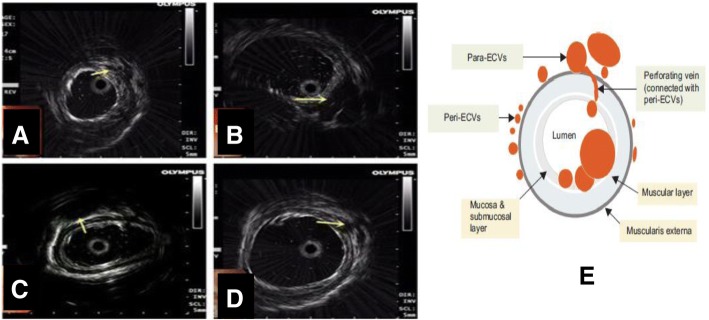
Endoscopic ultrasonography images of the esophagus. Enodscopic ultrasonography images were shown. a-b: the mild(a) and serious(b) peri-ECV(arrow), c: serious para-ECV(arrow), d: PFV(arrow). The diameter of varicose vein was also measured. Figure 2e from Gut Liver 2017;11:843–851
